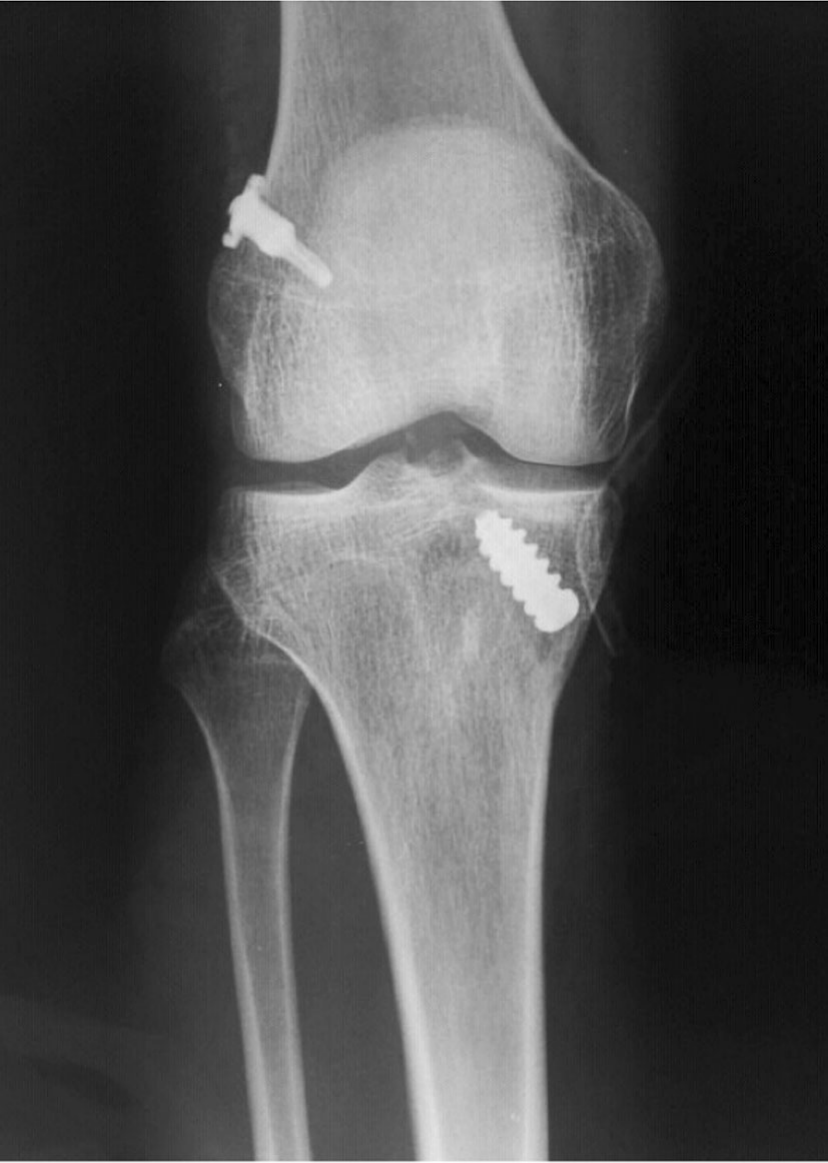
Postoperative anteroposterior radiograph of the right knee subjected to ACL reconstruction, showing femoral fixation with the ETD^®^
